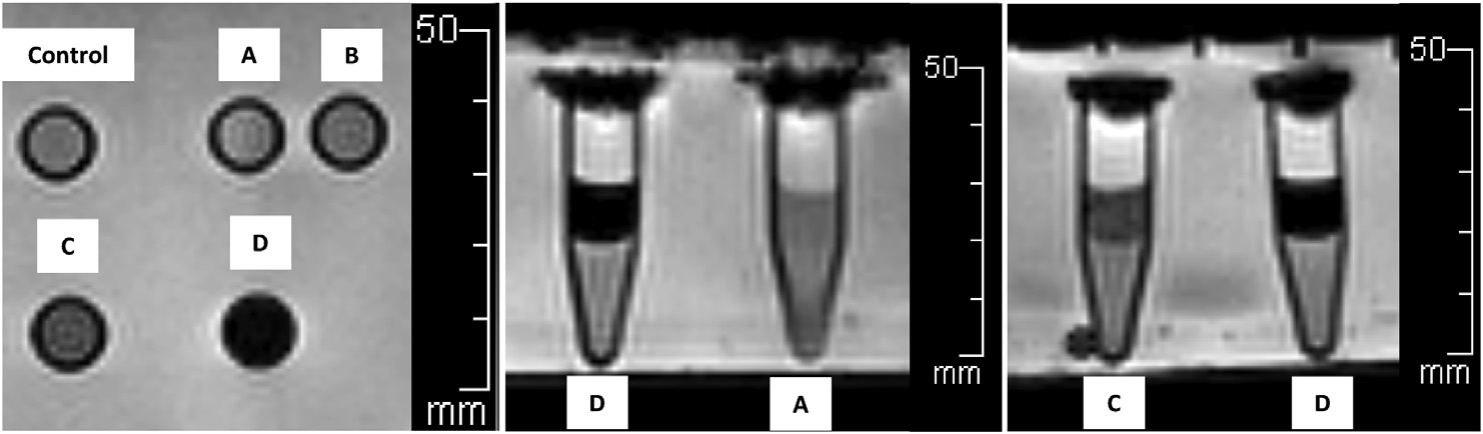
IS-labeled ASCs from T2∗-weighted sequences of 3T MRI. Left: Axial view, Middle: Sagittal view, Right: Coronal view. A: 1 × 10^3^ cells/ml/microtube, B: 1 × 10^4^ cells/ml/microtube, C: 1 × 10^5^ cells/ml/microtube, D: 1 × 10^6^ cells/ml/microtube.
